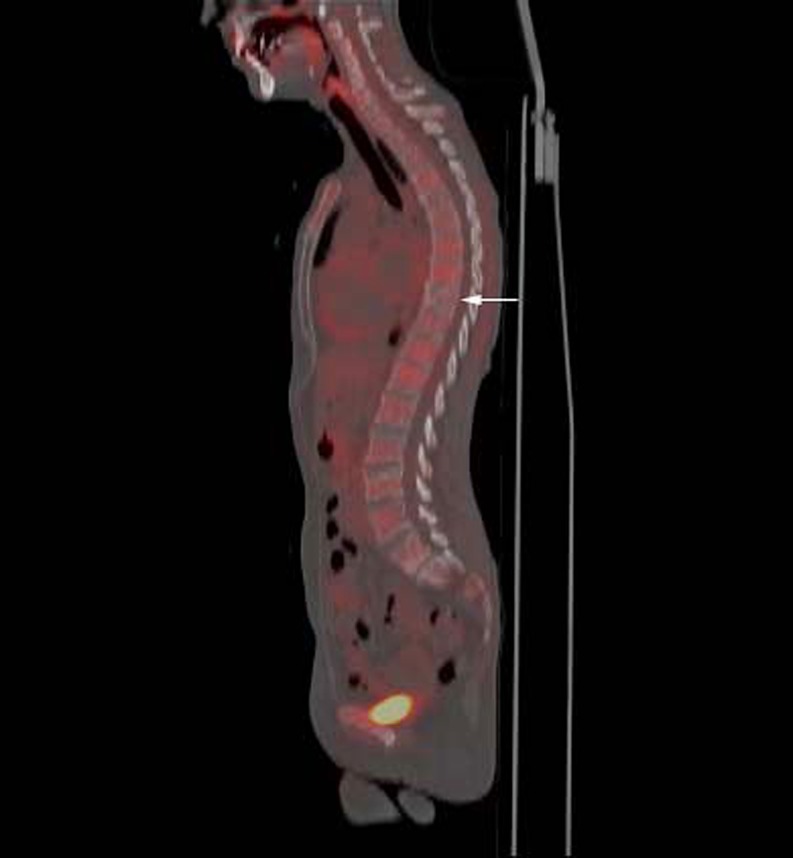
Benign compression fracture in a patient with lymphoma. Sagittal fused FDG-PET/CT image demonstrates compression fracture of T9 (*arrow*) without significant radiotracer uptake (SUV = 0.7)
